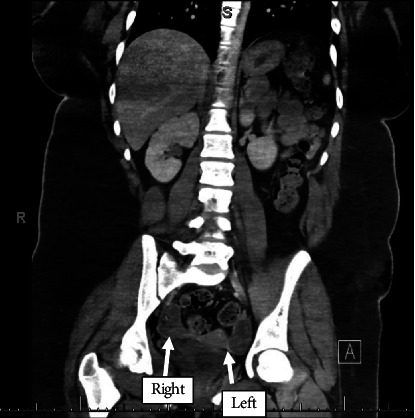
CT abdomen/pelvis demonstrating prominence of both ovaries including a small fat-containing area with punctate foci of adjacent hyperattenuation within the right ovary and an 8 cm^3^ ovoid area of relative hypoattenuation in the left ovary. Findings consistent with bilateral ovarian teratomas.
